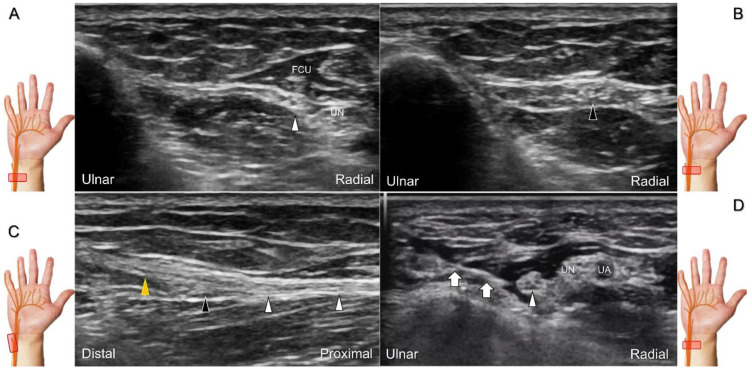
Sonographic tracking (short-axis view) of the dorsal ulnar cutaneous nerve from its normal (A) to the swollen segment (B) proximal to the entrapment. The normal, swollen, and entrapped segments of the dorsal ulnar cutaneous nerve are seen in long-axis imaging (C). Ultrasound-guided hydrodissection of the nerve (D). White arrowheads: normal segment; black arrowheads: swollen segment; orange arrowhead: entrapped segment; white arrows: needle. FCU: flexor carpi ulnaris muscle; UN: ulnar nerve; UA: ulnar artery.
